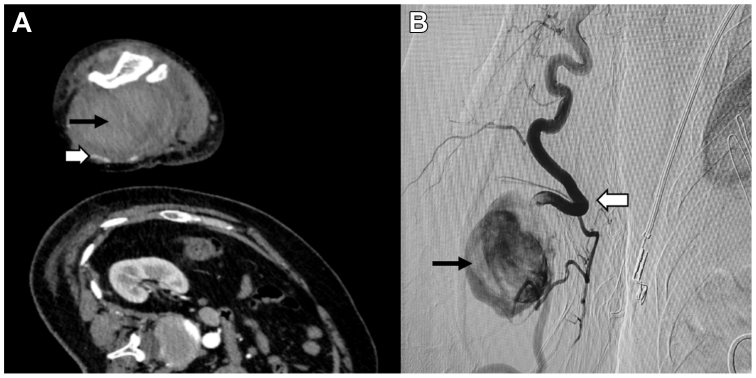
Computed tomography arteriography (CTA) and digital subtraction angiography (DSA) image of case 2. (A) A large hematoma (*black arrows*) can be seen on the patient's right upper arm. The right brachial artery was tortuous and was compressed by the hematoma (*white arrow*). (B) DSA image showing a tortuous brachial artery (*white arrow*) with contrast extravasation, indicating arterial rupture and pseudoaneurysm formation (*black arrow*).
